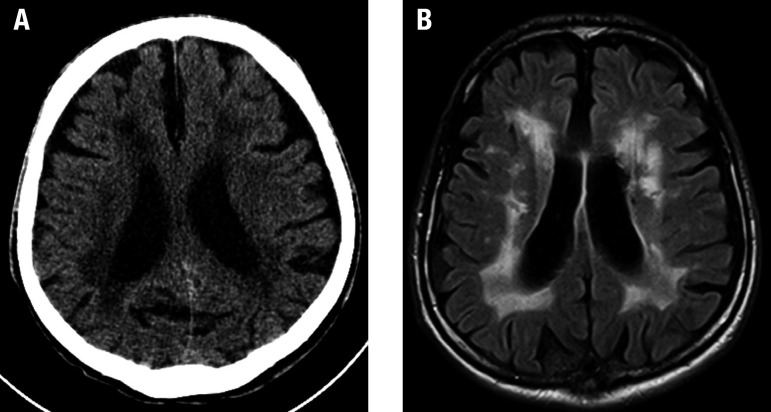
White matter hyperintensities of presumed vascular origin. An 80-year-old woman with cognitive impairment, hypertension and diabetes mellitus. Noncontrast CT image (A) shows diffuse and confluent hypoattenuation of cerebral white matter. Axial FLAIR image (B) demonstrates extensive white matter hyperintensities corresponding with CT hypoattenuation.
